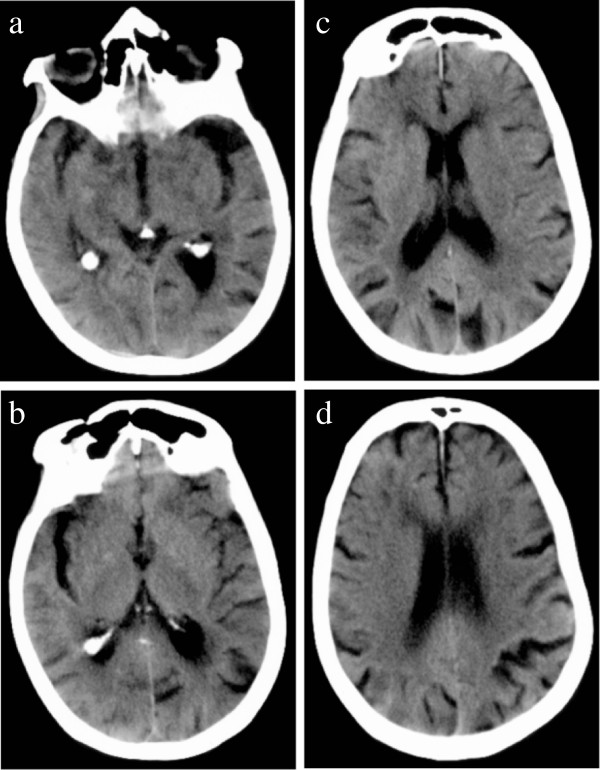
78 year old female in hypoglycaemic coma, computed tomography performed approximately 9 hours after onset of hypoglycaemia. There are areas of cortical low attenuation and swelling in the temporal and parietal lobes bilaterally.
